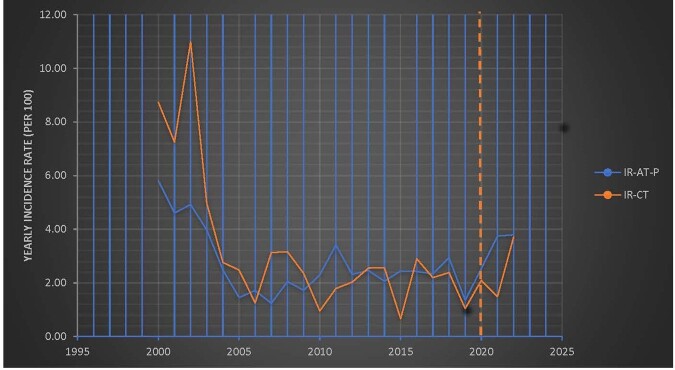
Incidence Rates of Congenital Toxoplasmosis (IR-CT)and Acute Toxoplasmosis During Pregnancy (IR-AT-P): 2000-2022
